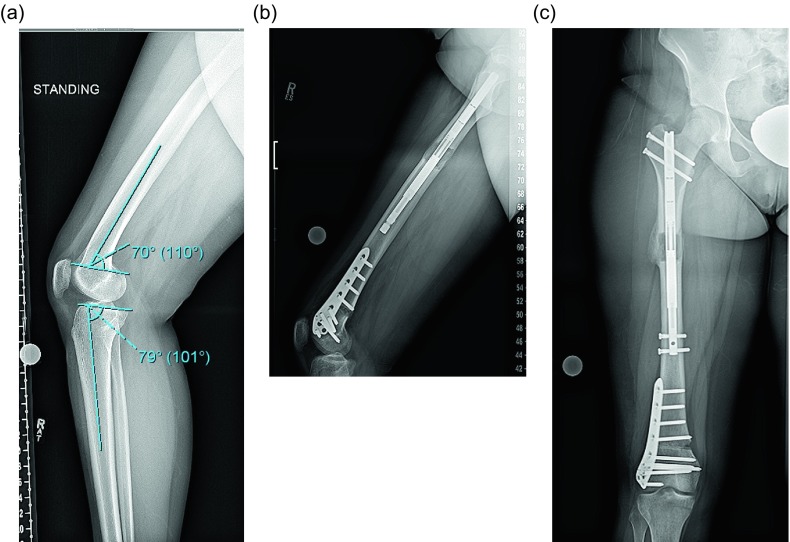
(a) This preop lateral radiograph shows a 13° flexion deformity of the distal femur. (b) Postop lateral shows a correction of the apex anterior deformity through an posterior opening wedge osteotomy stabilized with a plate and a proximal femoral lengthening with an ILN. (c) This far distal osteotomy would be difficult to control with an intramedullary implant.
